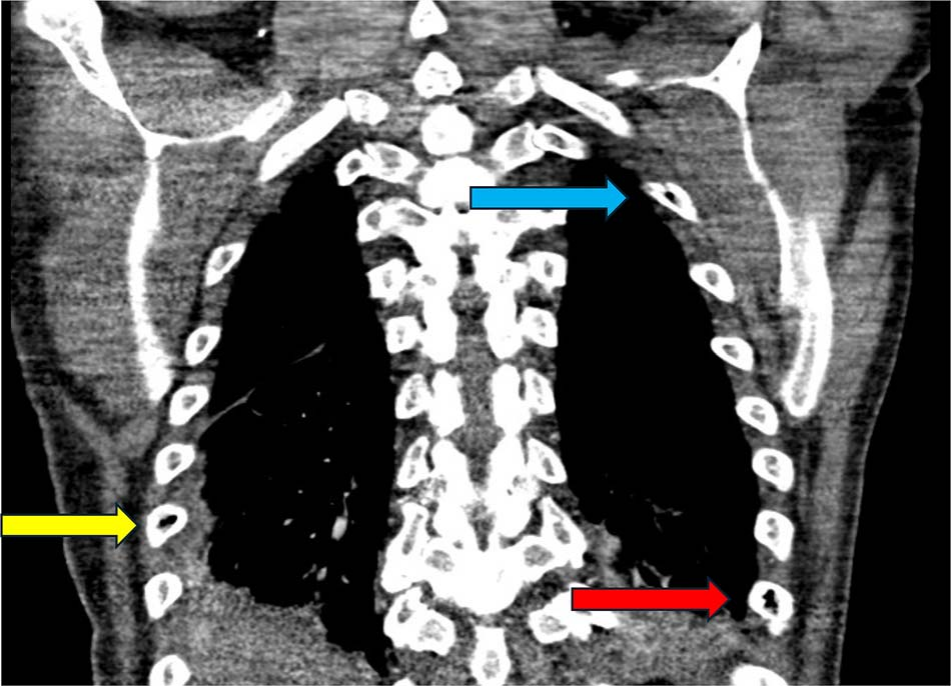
CT chest—coronal plane. Intraosseous gas is seen within the right sixth rib (yellow arrow, bottom left), left second rib (blue arrow, top right), and left eighth rib (red arrow, bottom right).
